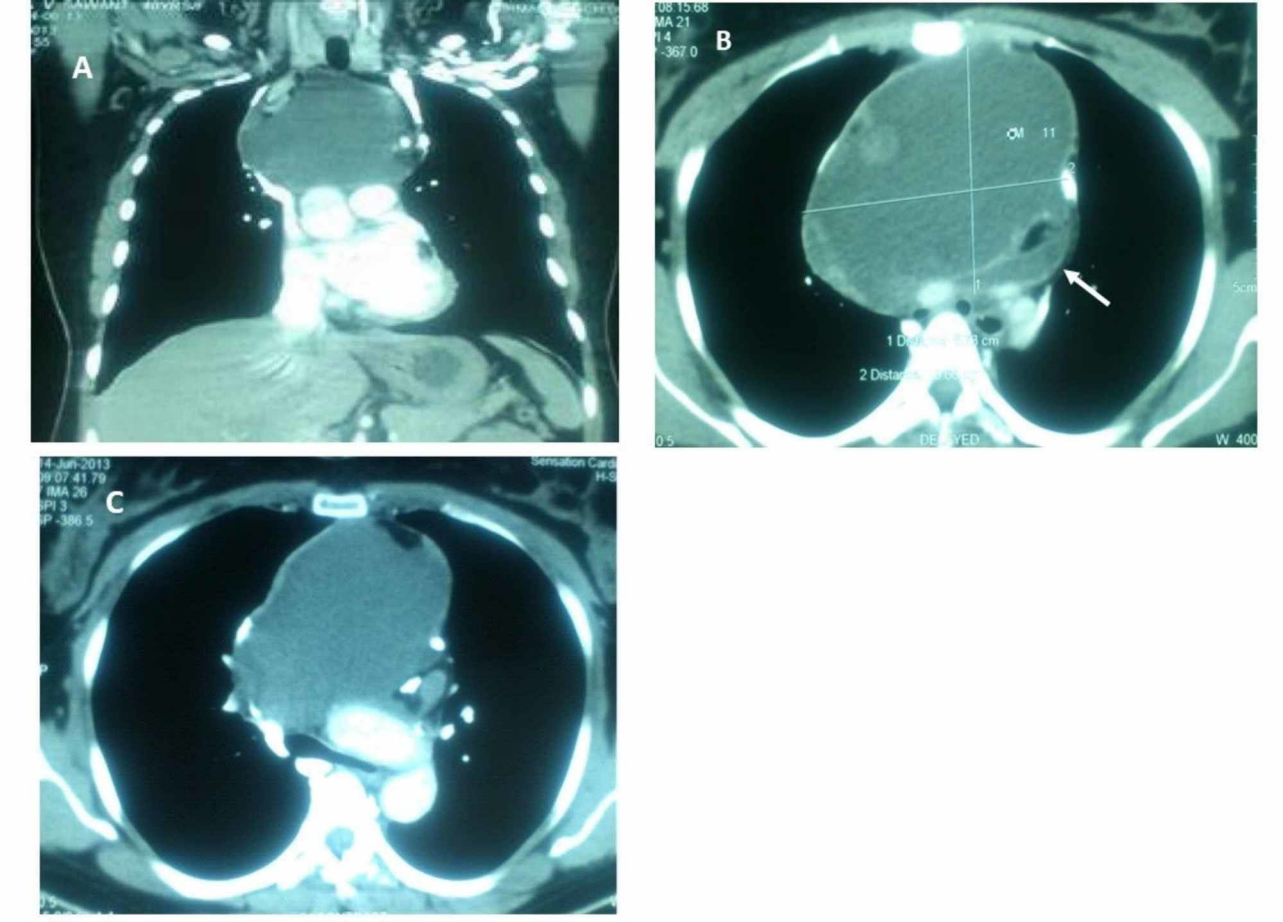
Computed tomography (CT) scan showing (A) tumor (white arrow) compressing the superior vena cava, and (B, C) trachea, right and left mainstream bronchus and arch of aorta
